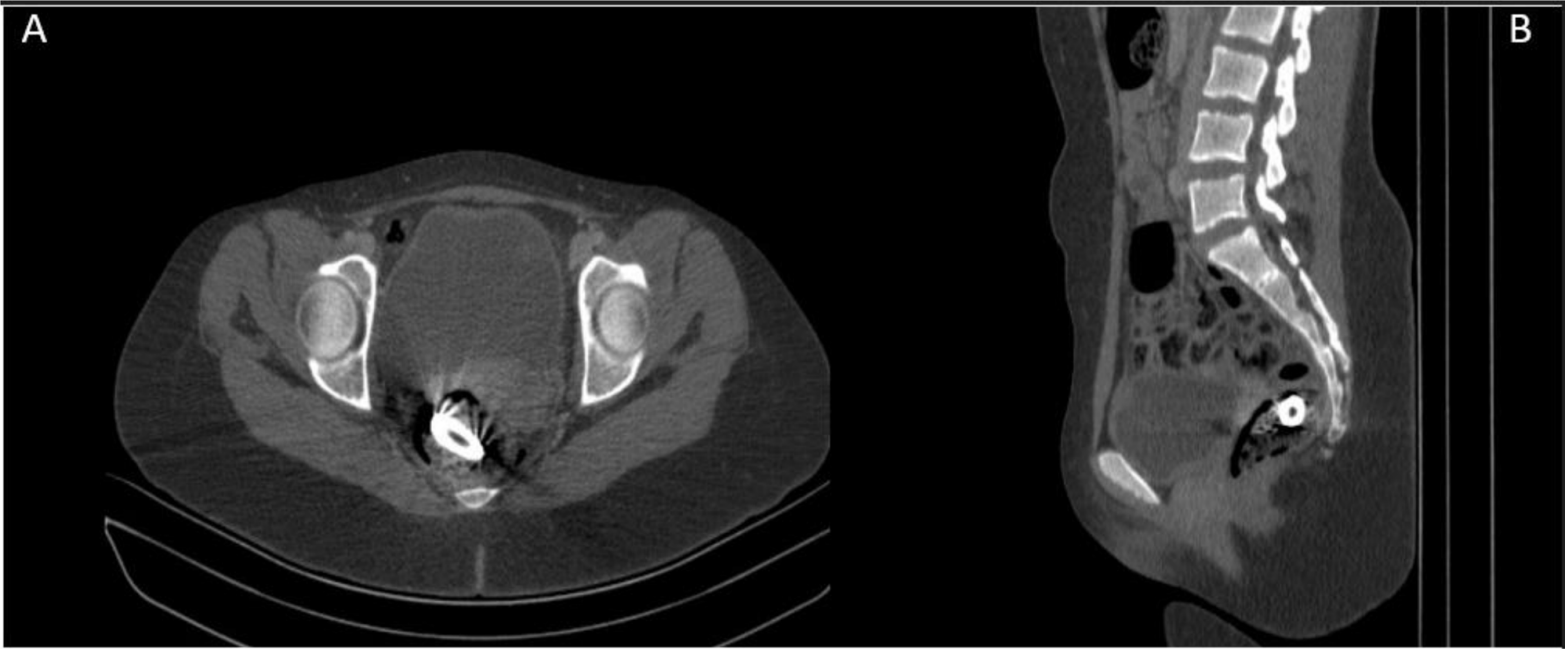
Axial (A) and sagittal (B) abdominopelvic CT images demonstrate a bullet shell casing lodged in the distal rectum, without evidence of bowel perforation
